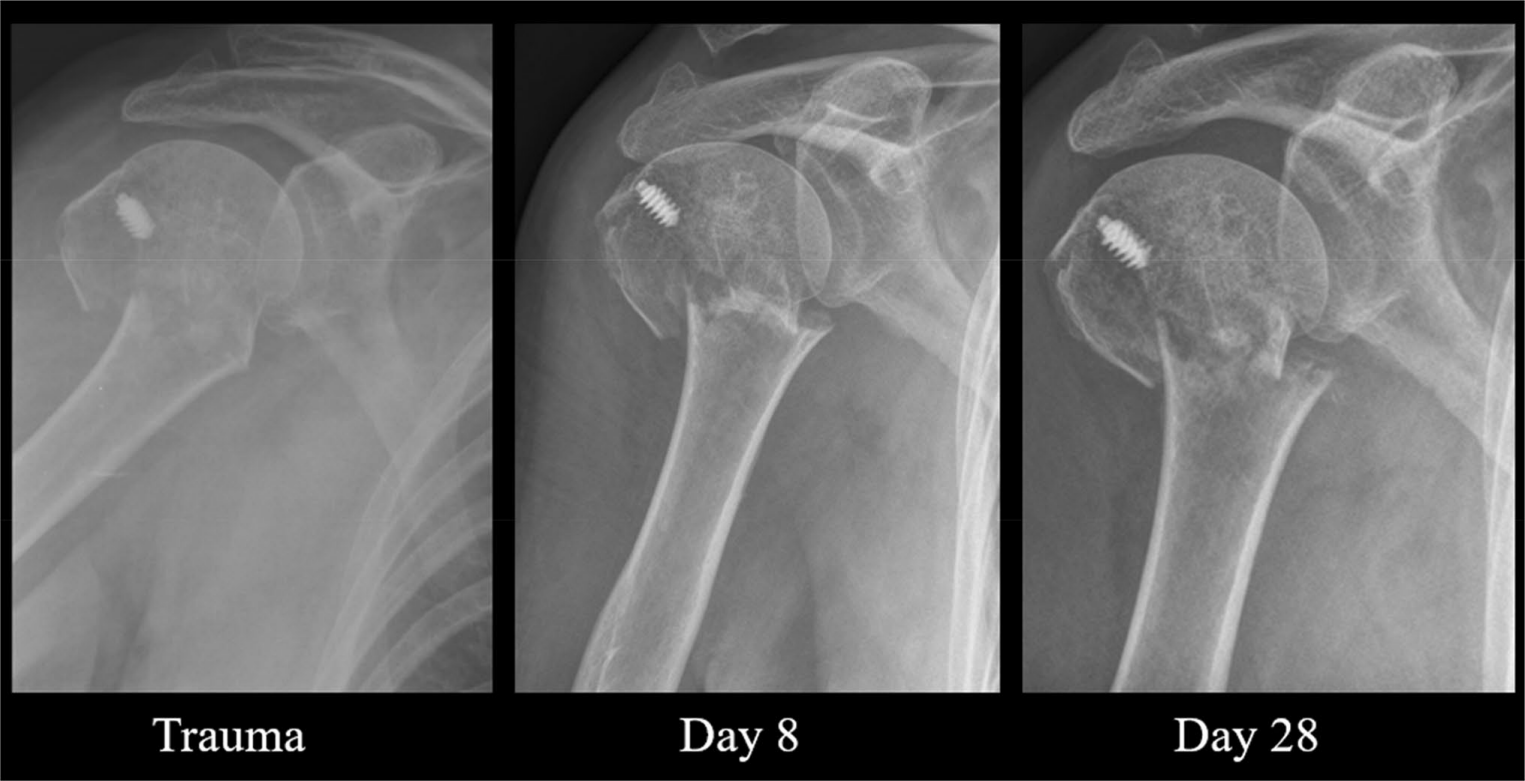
Radiographic follow-up of a type A fracture. Trauma: NSA = 178°, MMG = 5.6 mm, MLG = 10.1 mm. Day 8: NSA = 165°, MMG = 8.5 mm, MLG = 8.4 mm. Day 28: NSA = 161°, MMG = 10.3 mm, MLG = 11.2 mm
